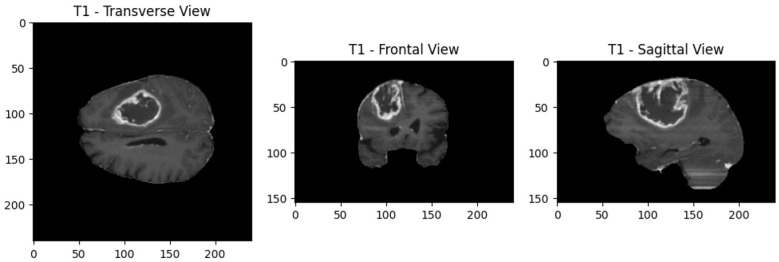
Viewing the MRI scans from different angles.
